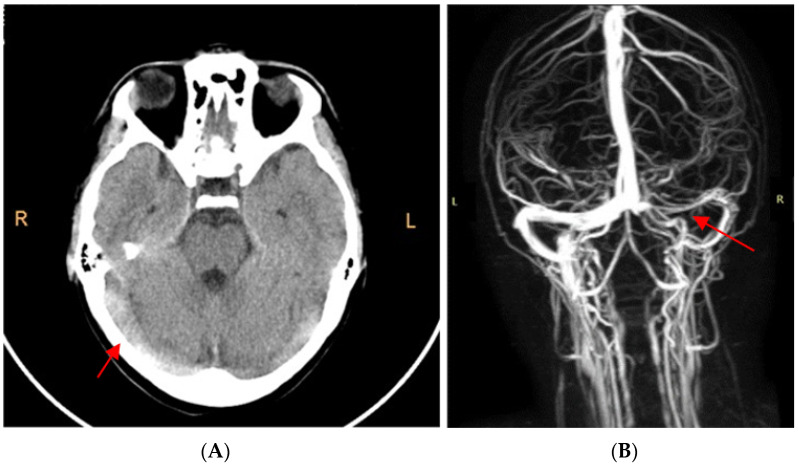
(A). CT scan without contrast: highlighted hyperdensity of the internal cerebral veins raised the suspicion of cerebral venous thrombosis. (B). MRI angiography: extended cerebral venous thrombosis affecting the right sigmoid sinus, both transverse sinuses, their point of confluence, and the right internal jugular vein.
